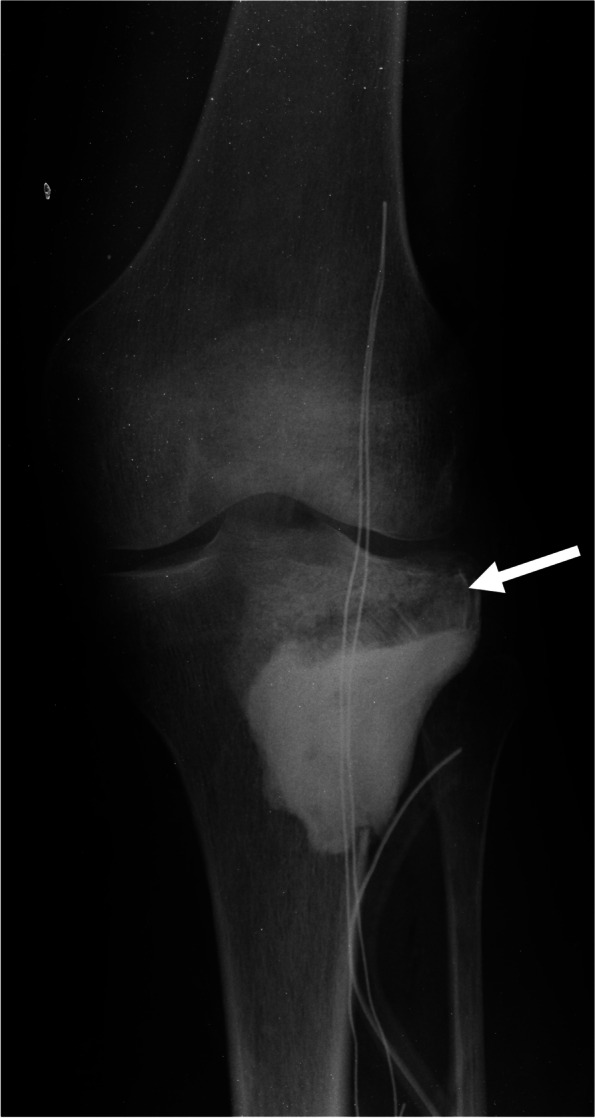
Radiography after PMMA bone cement filling following allograft chip bone filling in the subchondral region after curettage. The arrow indicates the allograft chip bone
